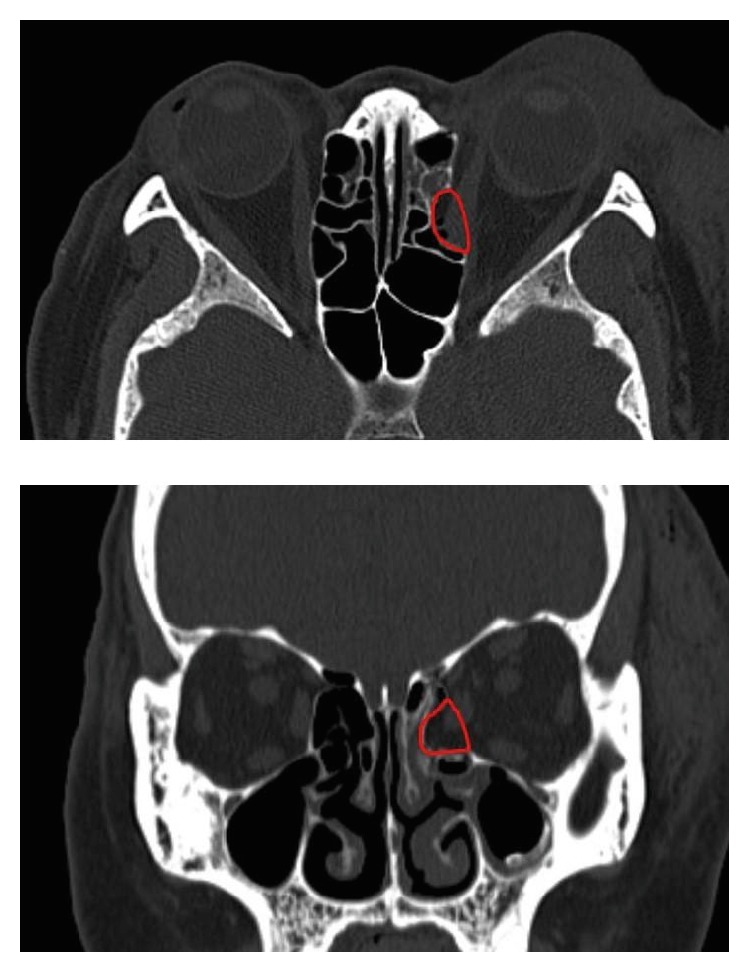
3D shape of the defect was estimated based on CT images. Red line indicates the defect area to be replaced by cancellous bone allograft with inlay implantation method.
